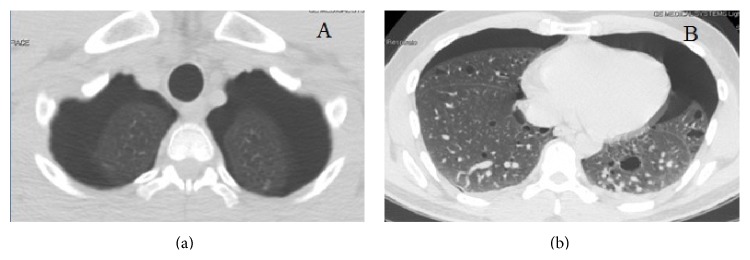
Chest CT-scan showing the apical (a) and the basal (b) aspect of the simultaneous bilateral spontaneous pneumothorax, as well as the multiple lung cysts (b).
